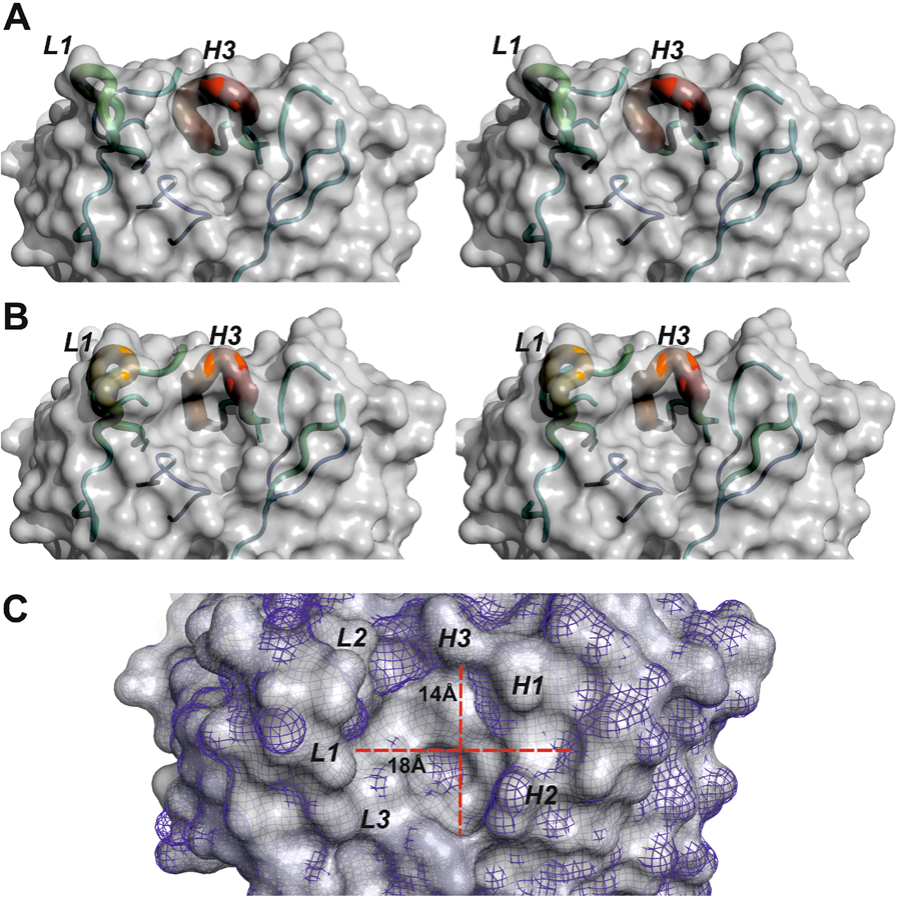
The flexibility of the binding site allows DC8E8 to adapt to four homologous, albeit not identical, structural determinants in the tau microtubule-binding repeats. Two independently refined X-ray structures of DC8E8 antigen-binding fragment (A) and (B) (stereoview) show the flexibility of the antigen-binding site. The surface of the antibody is shown in grey. The backbones of complementarity determining regions (CDRs) are represented as tubes, with the diameter and colour reflecting their averaged atomic displacement parameters, that is, flexibility. The flexibility is expressed as a colour scale ranging from blue to red, corresponding to B-factors 30 to 150 Å^2^. The CDRs L1 and H3 exhibit higher B-factors than the remaining parts of the model. The pronounced flexibility of these CDRs is essential to allowing DC8E8 to bind each of four slightly different epitopes within the microtubule-binding repeats (MTBRs). (C) Superposition of both independently refined DC8E8 molecules shows that the core of the binding pockets is invariant (molecule A shown as grey solid, molecule B as blue mesh). The CDR loops (italic letters) create a 7- to 9-Å-deep pocket with surface dimensions 18 × 14 Å (red axes). The shape of this pocket necessitates that the minimal DC8E8 epitope HXPGGG adopts a fold protruding into this space to bind in the DC8E8 combining site.
